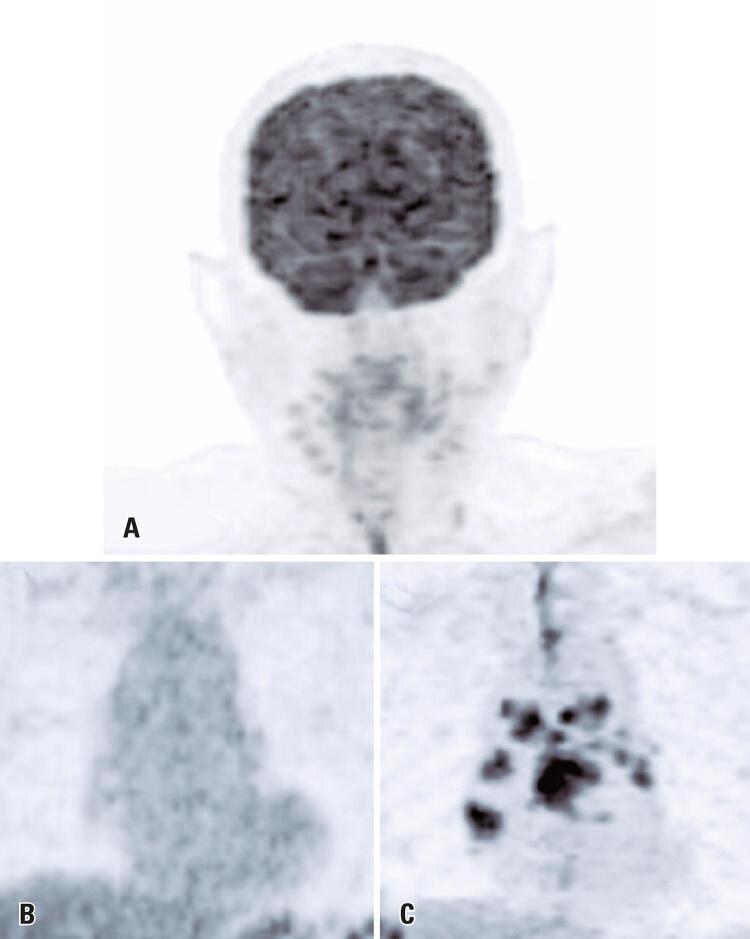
(A) PET-CT performed six weeks after one dose of cemiplimab showing an excellent partial response; (B) baseline PET-CT showing no mediastinal lymph nodes with FDG uptake; (C) PET-CT performed six weeks after one dose of cemiplimab showing a sarcoid-like reaction in the mediastinal lymph nodes
